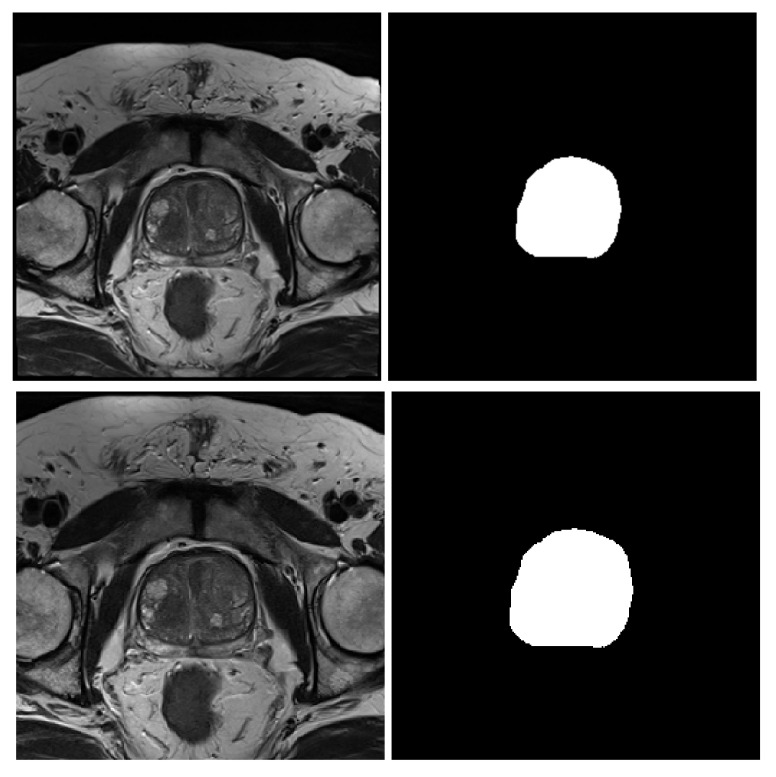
Center-cropping of MRI prostate image, original MRI (top-left), center-cropped image (bottom-left), and the corresponding masks (top/bottom-right).
